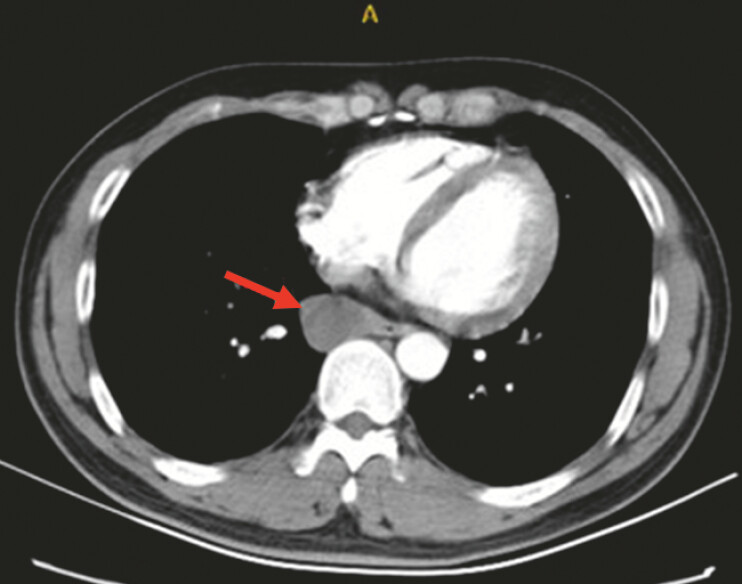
Enhanced computed tomography scan showing a hypodense mass of homogeneous density adjacent to the lower esophagus, about 3.0 cm in diameter, poorly demarcated from the esophagus and mediastinum, with unremarkable enhancement.
